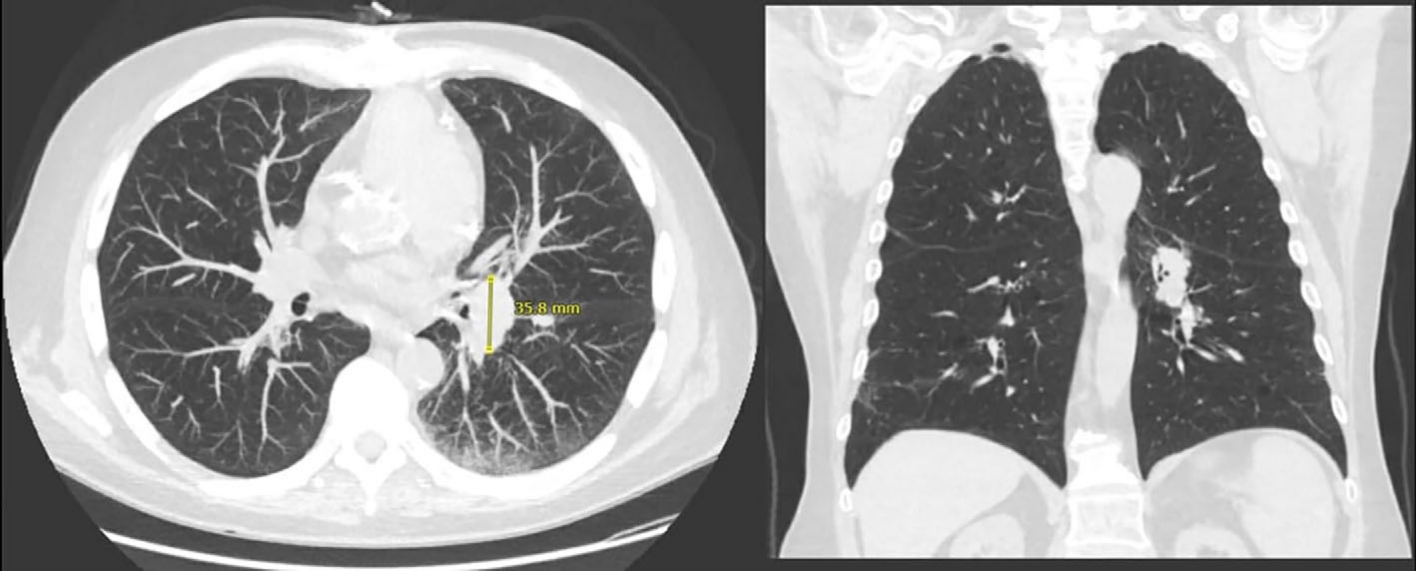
Transverse and coronal view of chest CT showing pulmonary nodule prior to COVID vaccine
